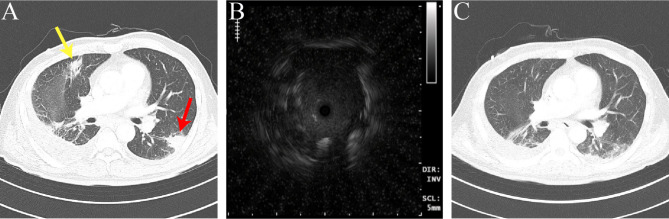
(A) An initial transverse CT scan reveals the presence of airspace consolidations measuring 2.1 and 2.2 cm-sized in the right upper lobe(yellow arrow) and left lower lobe(red arrow), respectively. A biopsy was performed on the consolidative lesion in the left lower lobe(red arrow). (B) Radial probe endobronchial ultrasound (RP-EBUS) image showing a concentric oriented lesion (‘within, concentric’ orientation) in consolidative lesion of left lower lobe. (C) Follow up CT scan after 2 cycles of Decitabine with Venetoclax demonstrates the disappearance of previous consolidative lesion
